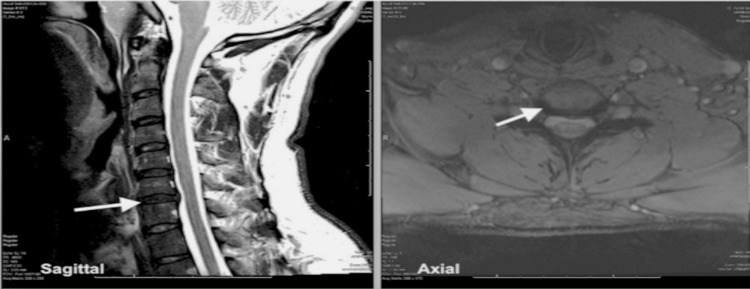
T2 Sagittal and Axial Views of the Cervical Spine and the C6-C7 level on MRI. A broad-based disc bulge at the level of C6-C7 is noted without neuroforaminal or central stenosis. No other acute pathology is noted on imaging. Left: T2 Sagittal View of Cervical Spine on MRI. Right: Axial View of Cervical Spine at the level of C6-C7 on MRI.
